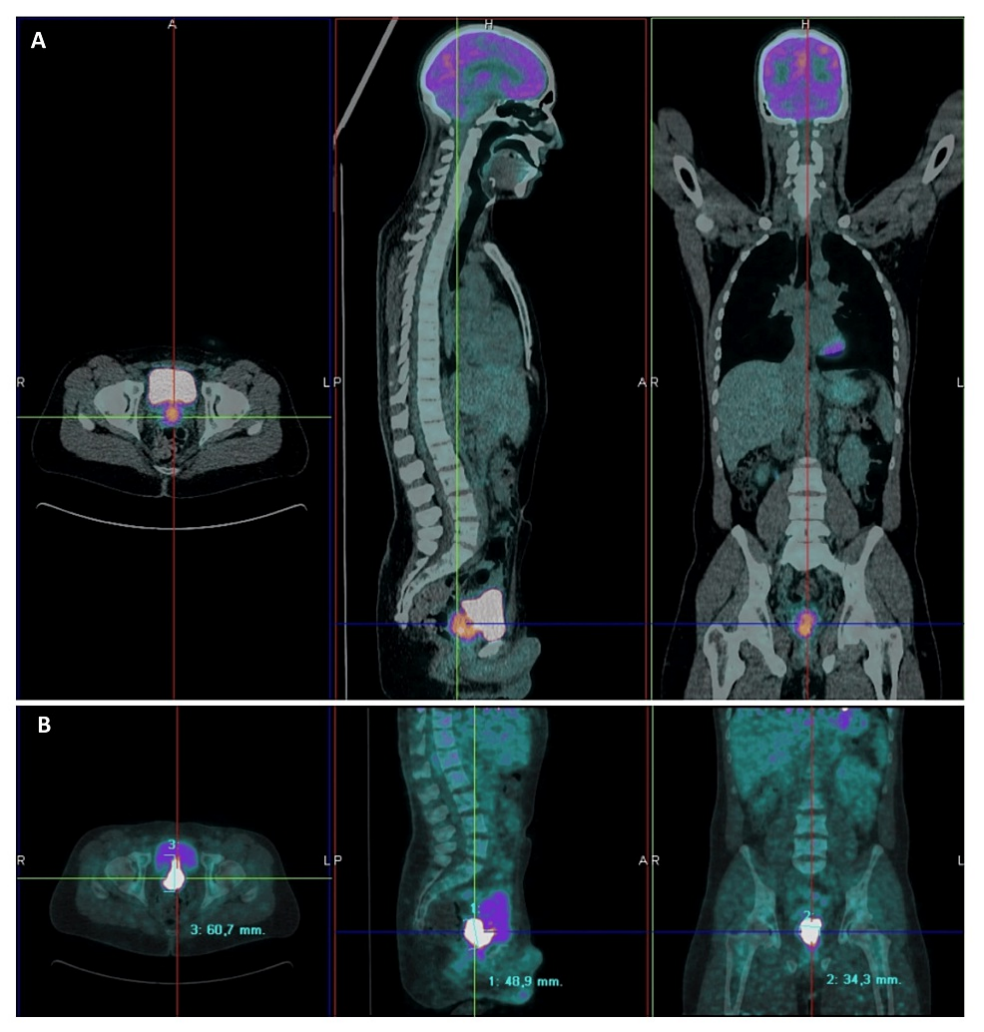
PET-CT with a large focus on uptake involving the anterior rectal wall, posteroinferior bladder wall, and the basis of both seminal vesicles, 49x61x34mm, (A) SUV 18.87 and (B) 21 in later images. PET-CT: positron emission tomography CT; SUV: standardized uptake value
